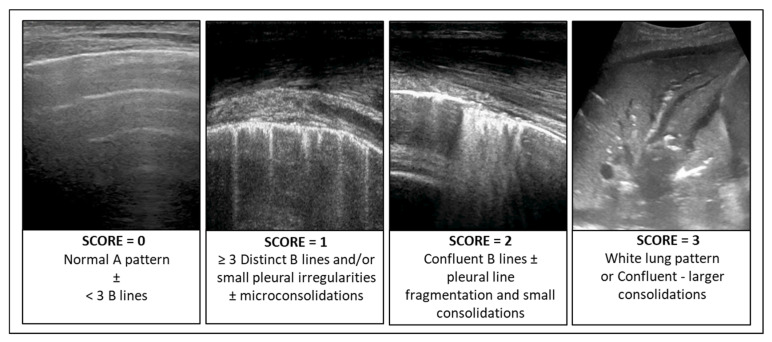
Ultrasound findings for calculating the Lung Ultrasound Score (LUSsc 0–36).
